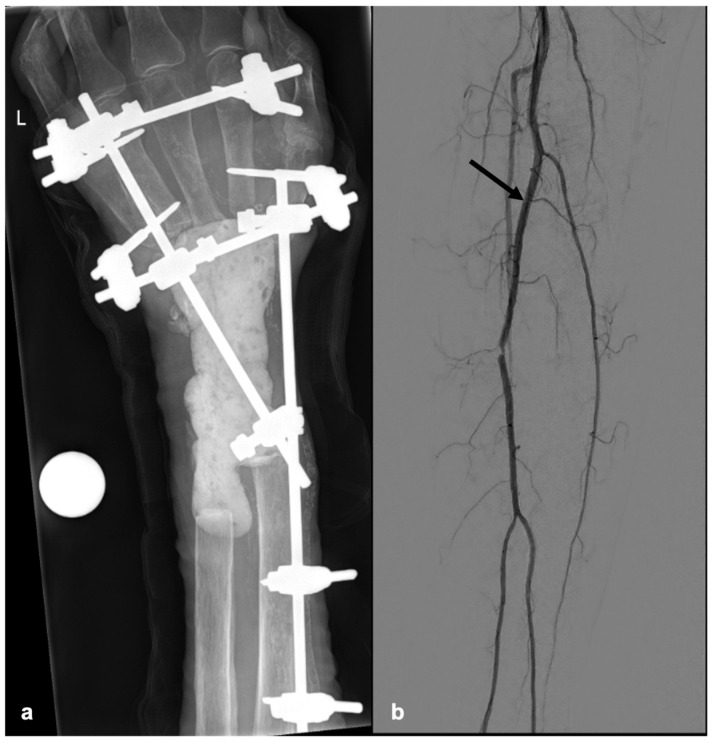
Preoperative planning. (a) X-ray of the wrist with extensive bone defect and local cement spacer and external fixation. (b) Lower-extremity arteriography with a peronea magna artery (black arrow) and severe arteriosclerosis.
